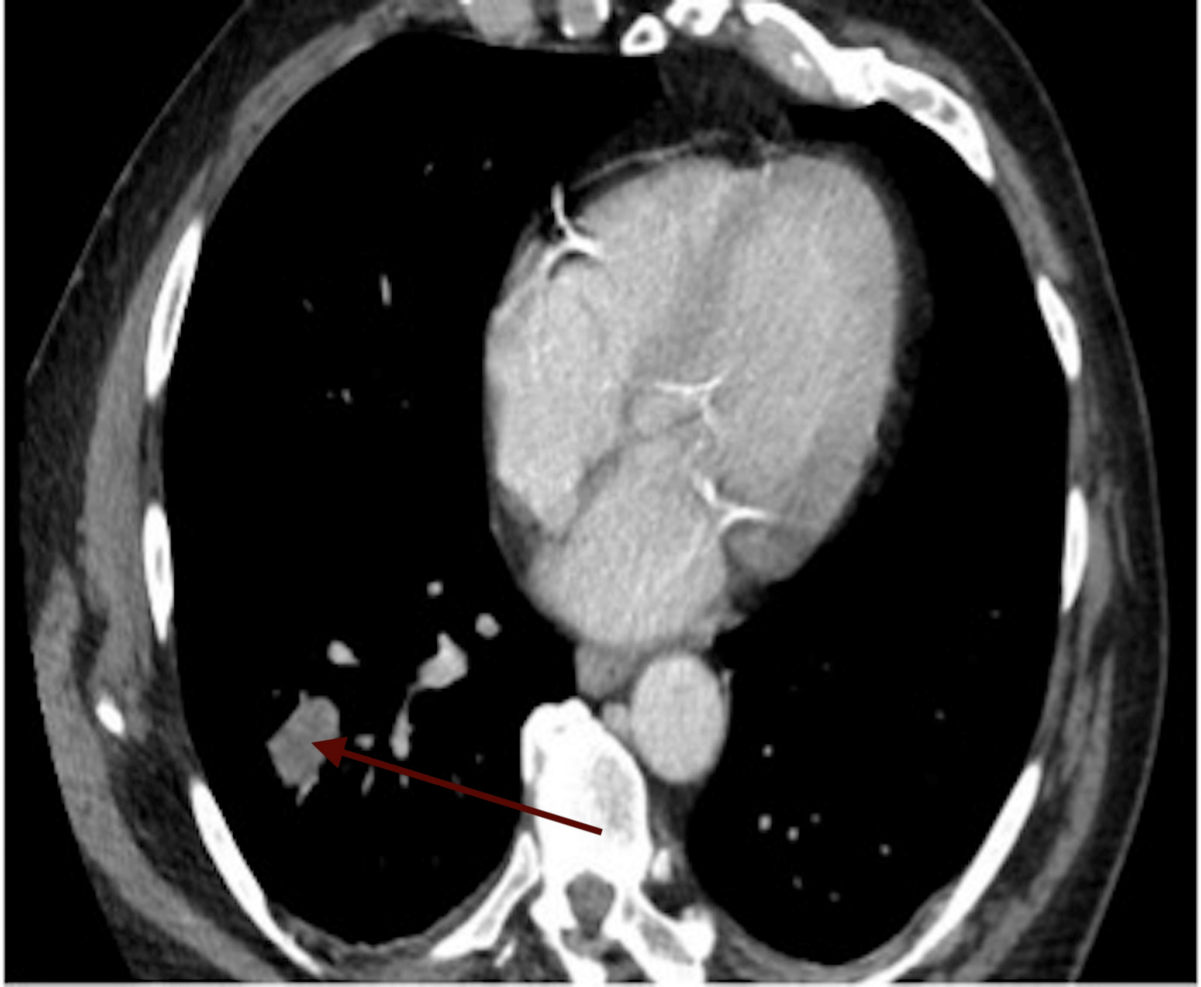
CT image of the primary lesion incidentally found on additional imaging The red arrow depicts a malignant lesion of the right lung. CT: Computed tomography
